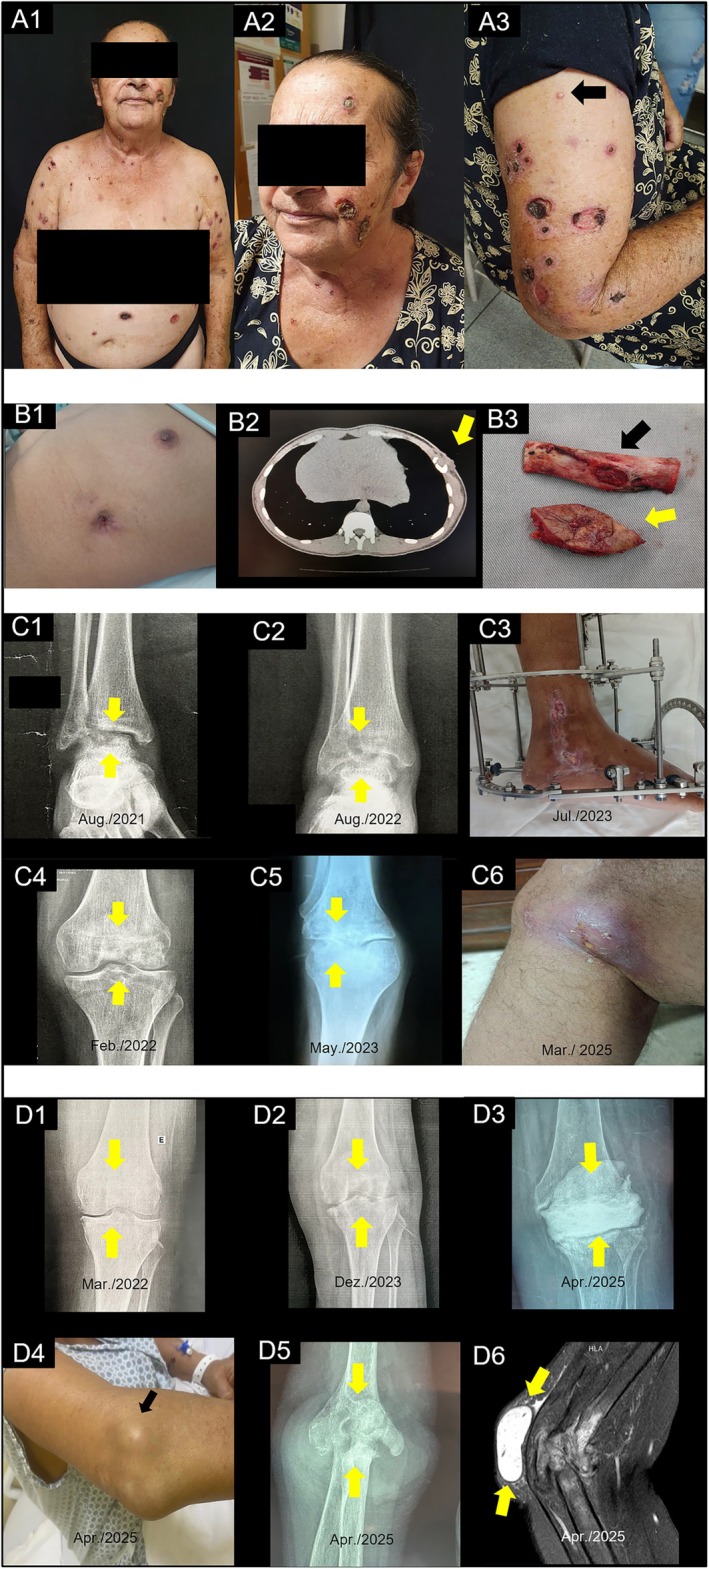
Representative pictures of OS patients. *Case 7*: Widespread nodular lesions throughout the body. (A1–A3) Lesions at different stages of progression and a new lesion (black arrow). *Case 20*: Osteomyelitis in the left 7th costal arch. (B1) Single slow‐healing sporotrichosis lesion. (B2) Chest computed tomography (CT) showing an osteolytic, permeative lesion with a periosteal reaction (yellow arrow). (B3) Bone fragment with osteolytic lesion (black arrow) and skin fragment (yellow arrow). *Case 16*: Multifocal OS. (C1) Right ankle arthritis with early joint space narrowing, bone marrow edema, and abscesses. (C2) One‐year follow‐up showing progression to osteomyelitis with joint space narrowing and extensive bone erosions. (C3) Ankle arthrodesis. (C4) Left knee arthritis with joint space narrowing and medial femoral and tibial erosions. (C5) Fifteen‐month follow‐up showing marked joint space narrowing and multiple large bone erosions. (C6) Draining skin lesions on the left knee. *Case 19*: Multifocal OS. (D1) Progressive left knee arthritis with joint space narrowing. (D2) Left knee osteomyelitis after 21 months, with narrowing and multiple erosions. (D3) Post‐surgery and orthopaedic cement in the left knee. (D4) Nodular lesion on the right elbow (black arrow). (D5) Progression to osteomyelitis of the left elbow after 57 months. (D6) Magnetic Resonance (MR) imaging showing a large amount of fluid located in the posterior region of the right elbow (yellow arrow).
